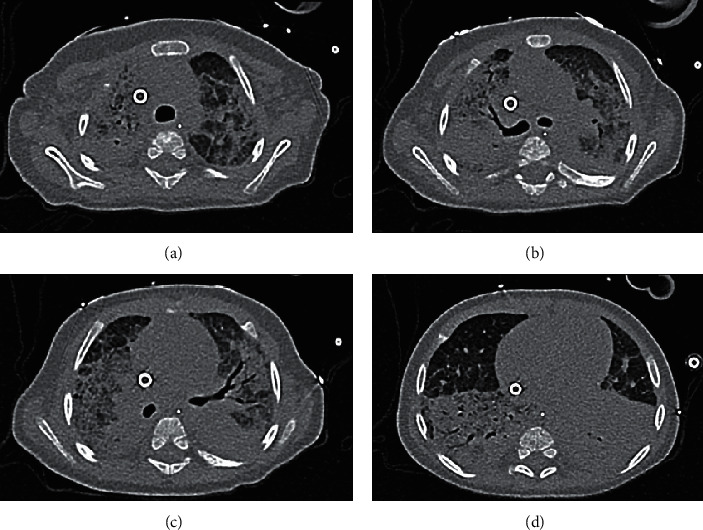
A thoracic computed tomograph taken during VV-ECMO-assisted recovery from *Pneumocystis* pneumonia-caused ARDS. (a) Apical lesions; (b) Carinal lesions associated with fibrosis and alveolar condensation; (c) lower lobe condensation (an air bronchogram); (d) lung base condensations. Note the Avalon Cannula in the vena cava in all panels.
